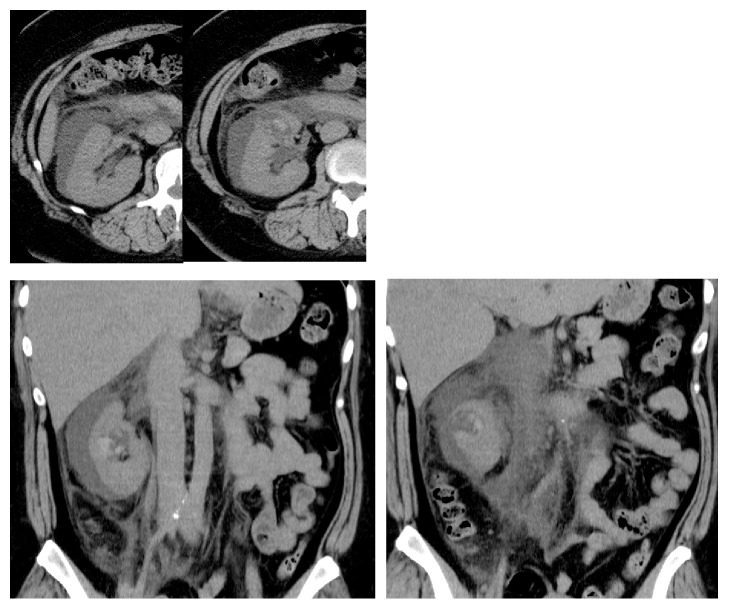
Computed tomography image on postoperative day 1 showing perinephric extravasation of urine and collapse of calyceal diverticulum. A high-density area of the diverticulum indicated bleeding due to rupture.
